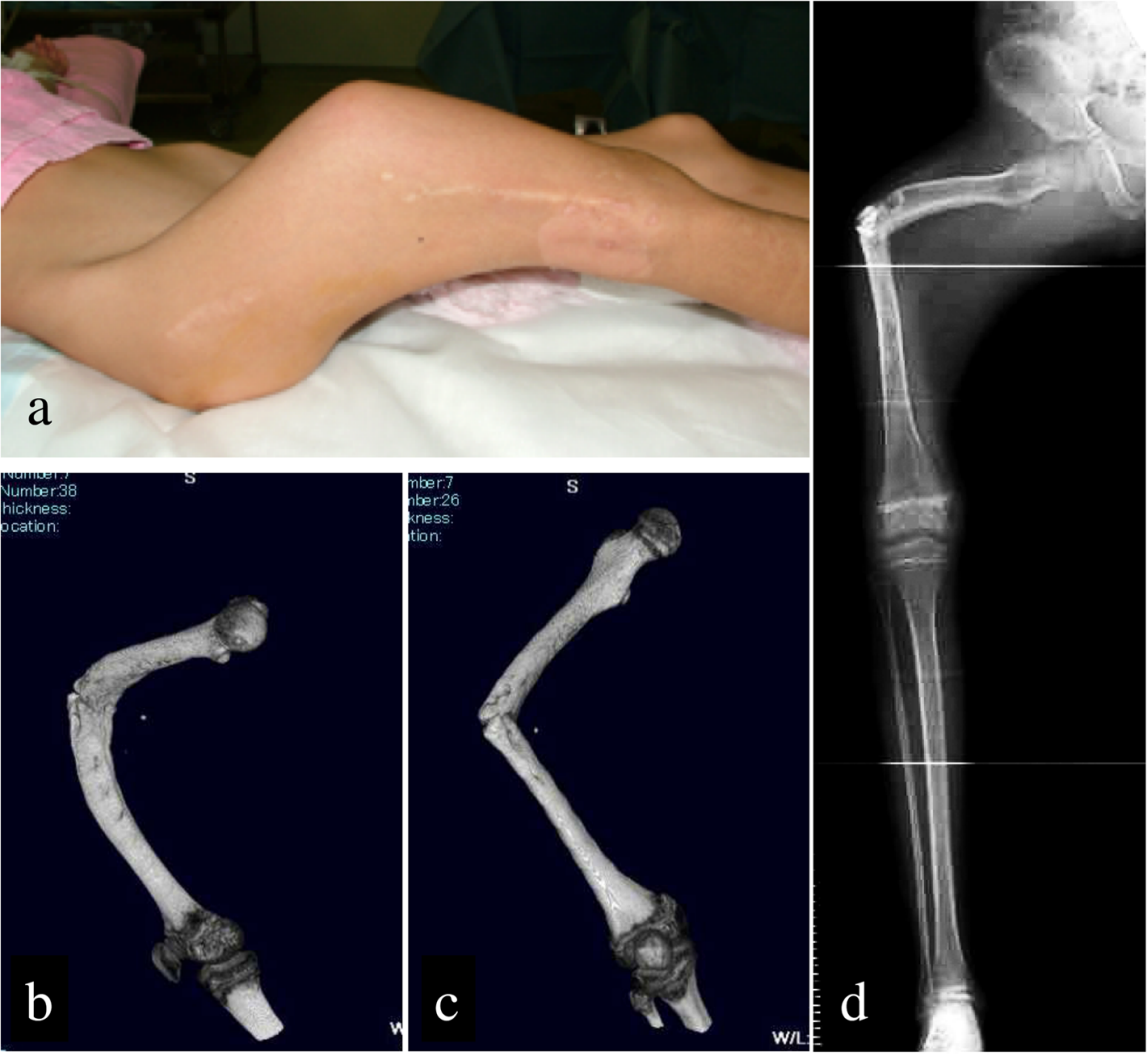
At age 9 years, 7 months upon visiting our facility. a External appearance of the right femur. b, c Right femur three-dimensional computed tomography. d Simple radiograph of the entire right lower limb. Upon first examination at our facility, the right femur was deformed, with bowing of approximately 90° and internal rotation of 60° with incomplete fractures in the same area
